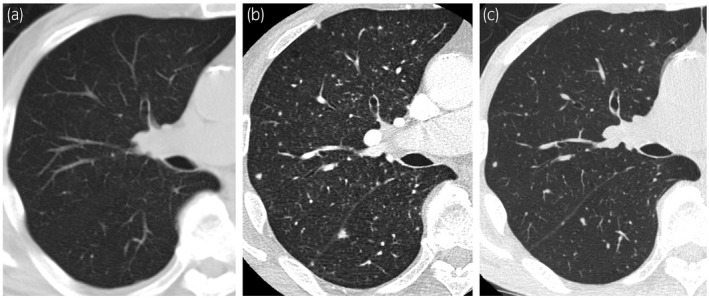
Chest CT findings. (a) No findings on the postoperative day 4. (b) Diffuse granular shadows were observed in bilateral lung fields, which was a typical finding of miliary tuberculosis. (c) Improvement of granular shadows after 4 months of treatment.
